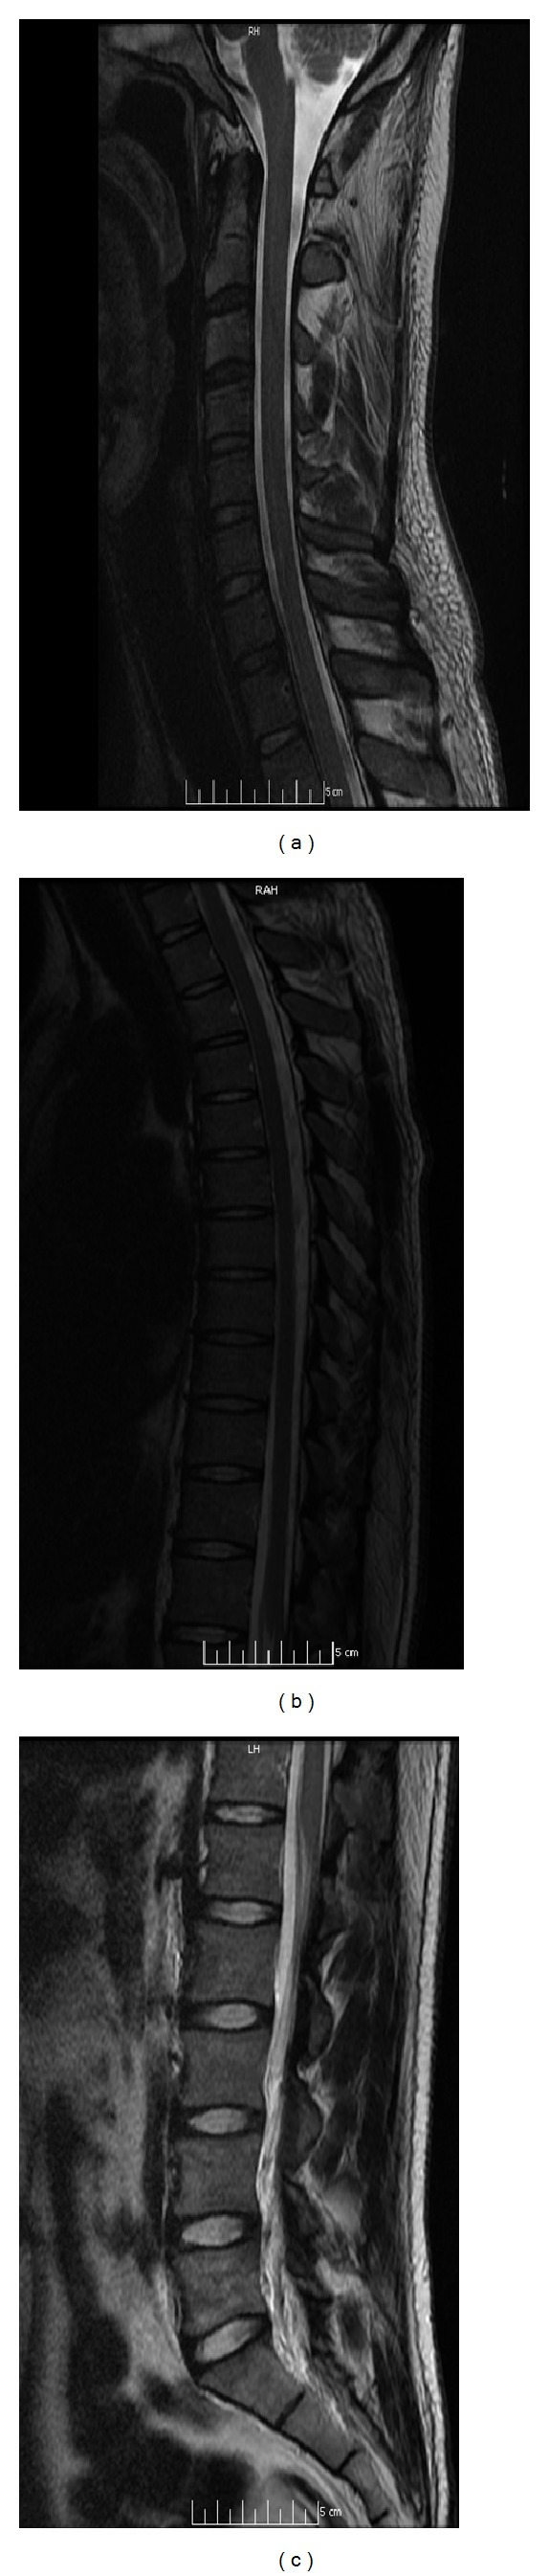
Sagittal MRI of the cervical, thoracic, and lumbosacral spinal cord (T2W; (a) to (c)) was unremarkable without a demonstrable compressive lesion.
